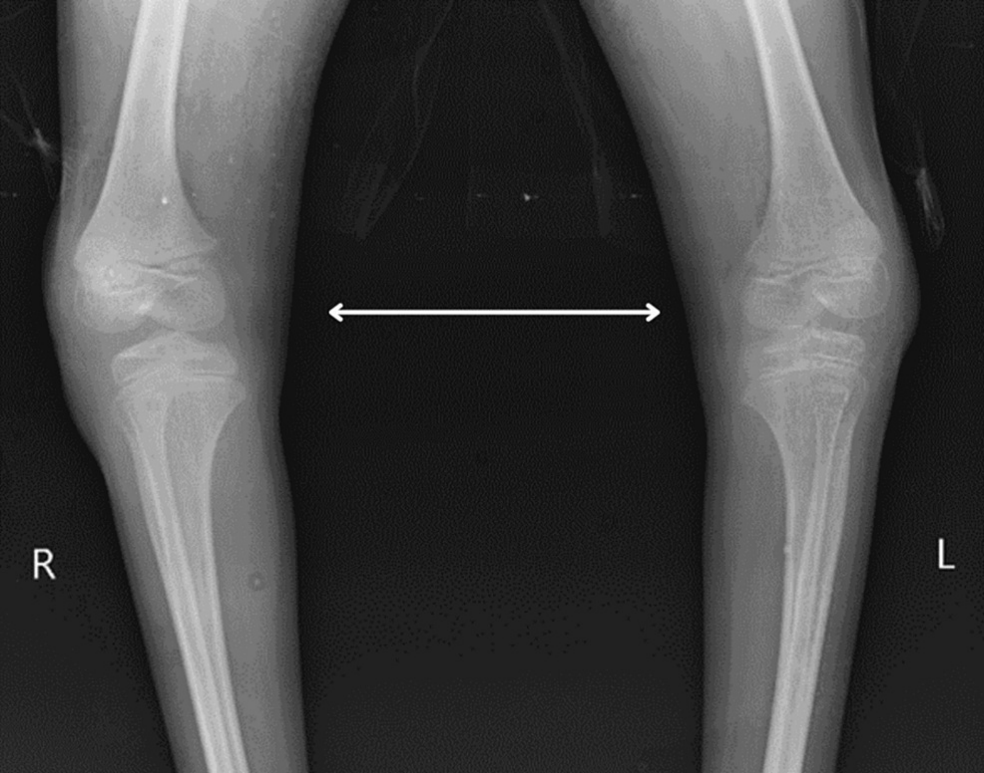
X-ray showing the leg bowing outward at the knee, while the lower leg is angled medially
